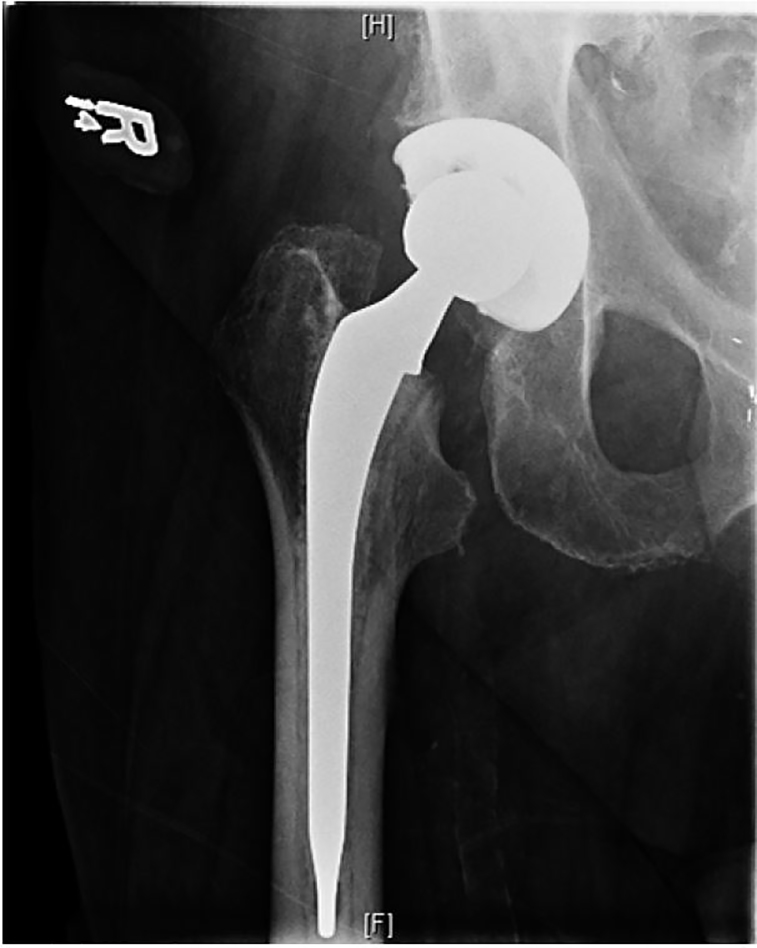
Post reduction anterior-posterior radiograph of the right hip demonstrating normal joint alignment.
